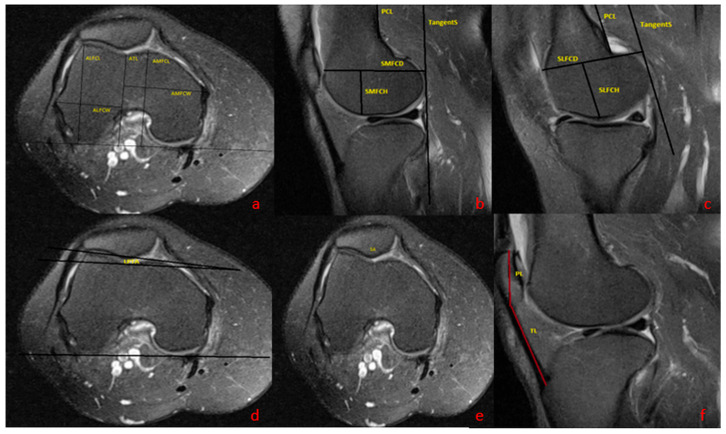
(a). Length measurements on axial MRI, (b). Medial condyle parameters on sagittal MRI, (c). Lateral condyle parameters on sagittal MRI, (d). LPTA measurement, (e). Sulcus angle measurement, (f). Insall-Salvati ratio measurement.
